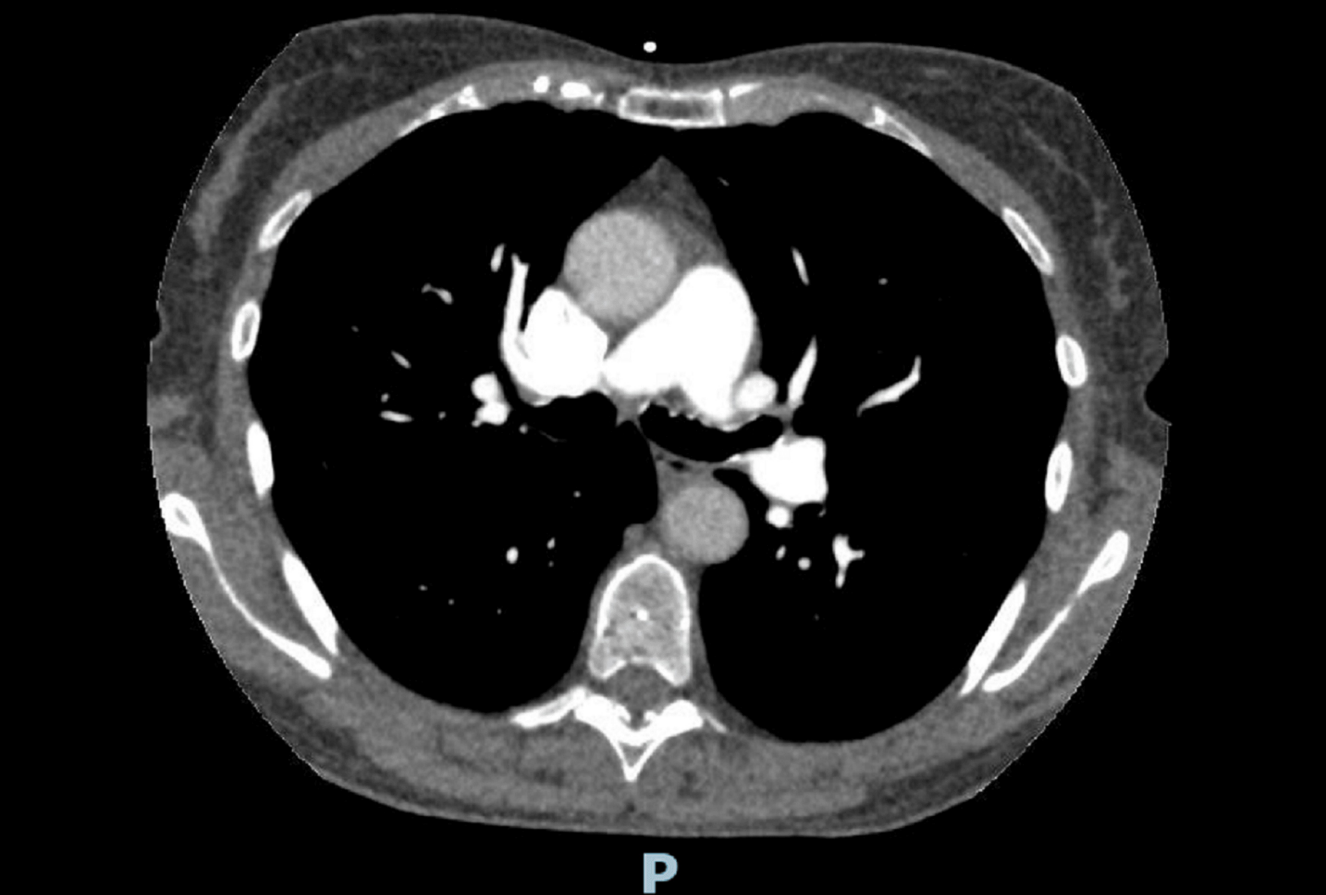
CT pulmonary angiogram (CTPA) CTPA did not show any evidence of pulmonary embolus
